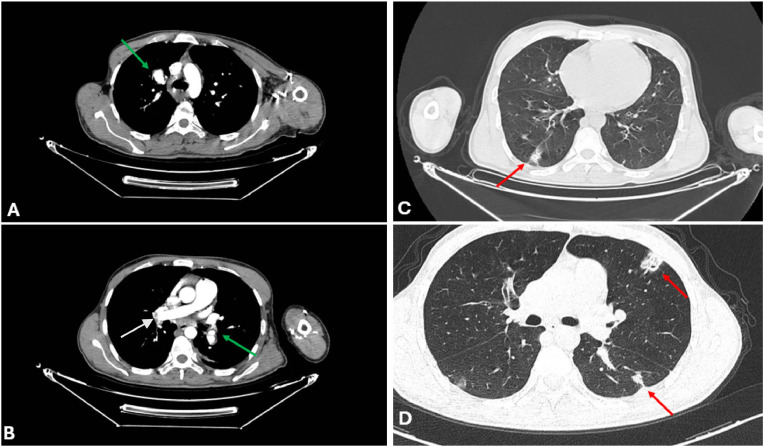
Chest angiography CT scan revealing pulmonary arterial anevrysms images A and B (green arrow), and hemorrhagic alveolar foci (red arrow) with pulmonary arterial embolism (white arrow).
